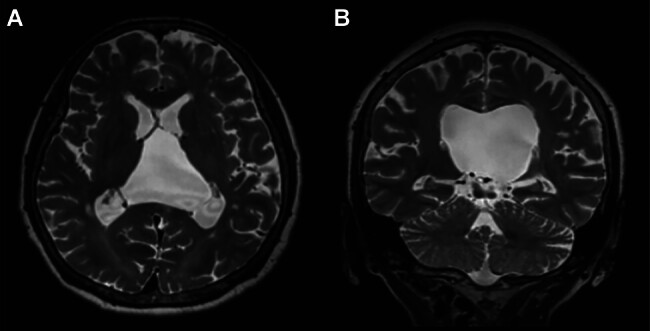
Postoperative MRI. (A, B) Head MRI taken 3 months after surgery. The cyst decreases, and the lateral ventricle increases in size. MRI, magnetic resonance imaging.
